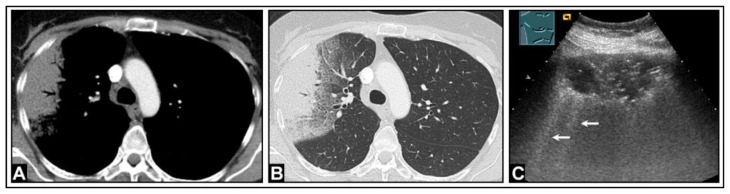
A patient with fever, auscultatory crackles, elevated inflammatory markers, and clinical suspicion of community-acquired pneumonia. (A,B) Computed tomography reveals consolidation with ground-glass opacities in the transitional area between consolidated and normal lung tissue. (C) Lung ultrasound demonstrates hypoechoic consolidation with air bronchogram; the border of the lesion to the lung appears blurred, with multiple partially confluent B-lines (arrows) originating from the interface.
